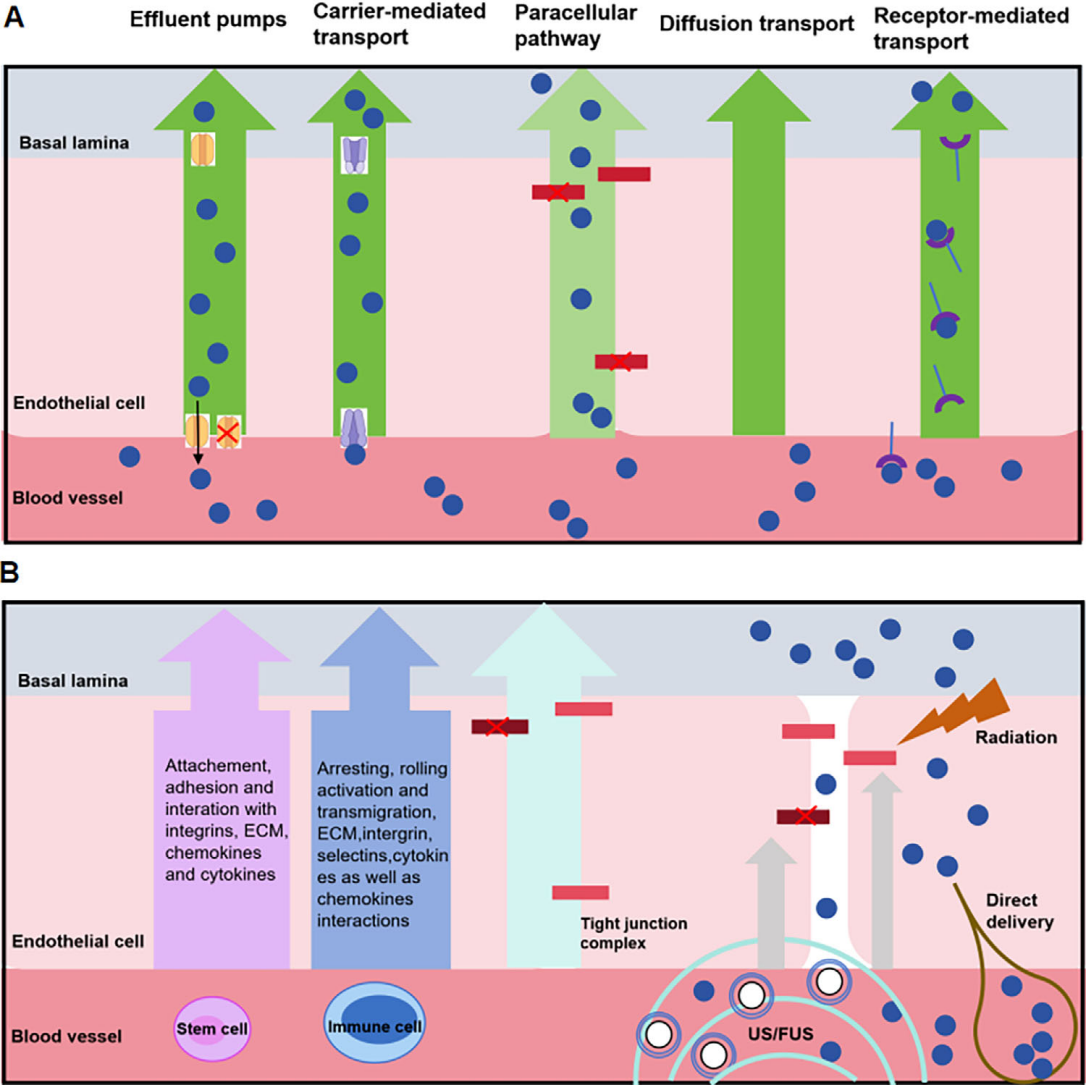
The multiple strategies/pathways are employed to overcome BBB. (A) The molecular strategies/pathways are applied to overcome BBB. The drugs or therapeutics agents (blue circles) can be designed with either affinity to efflux pumps or receptor/carrier‐mediated transport mechanisms as well as paracellular pathways by junctional complexes. (B) The cellular and direct delivery strategies/pathways to bypass BBB. The immune cells could transmigrate to the CNS mediated by actin‐containing protrusive structures. And also stem cells that have been modified and displayed transcellular or paracellular transmigration. Directly, ultrasound or focused ultrasound (US/FUS) with microbubbles (concentric small blue circles), radiation could mediate direct delivery of therapeutics to the CNS. Reproduced with the authors permission [186]. Copyright 2020. HHS Public Access.
